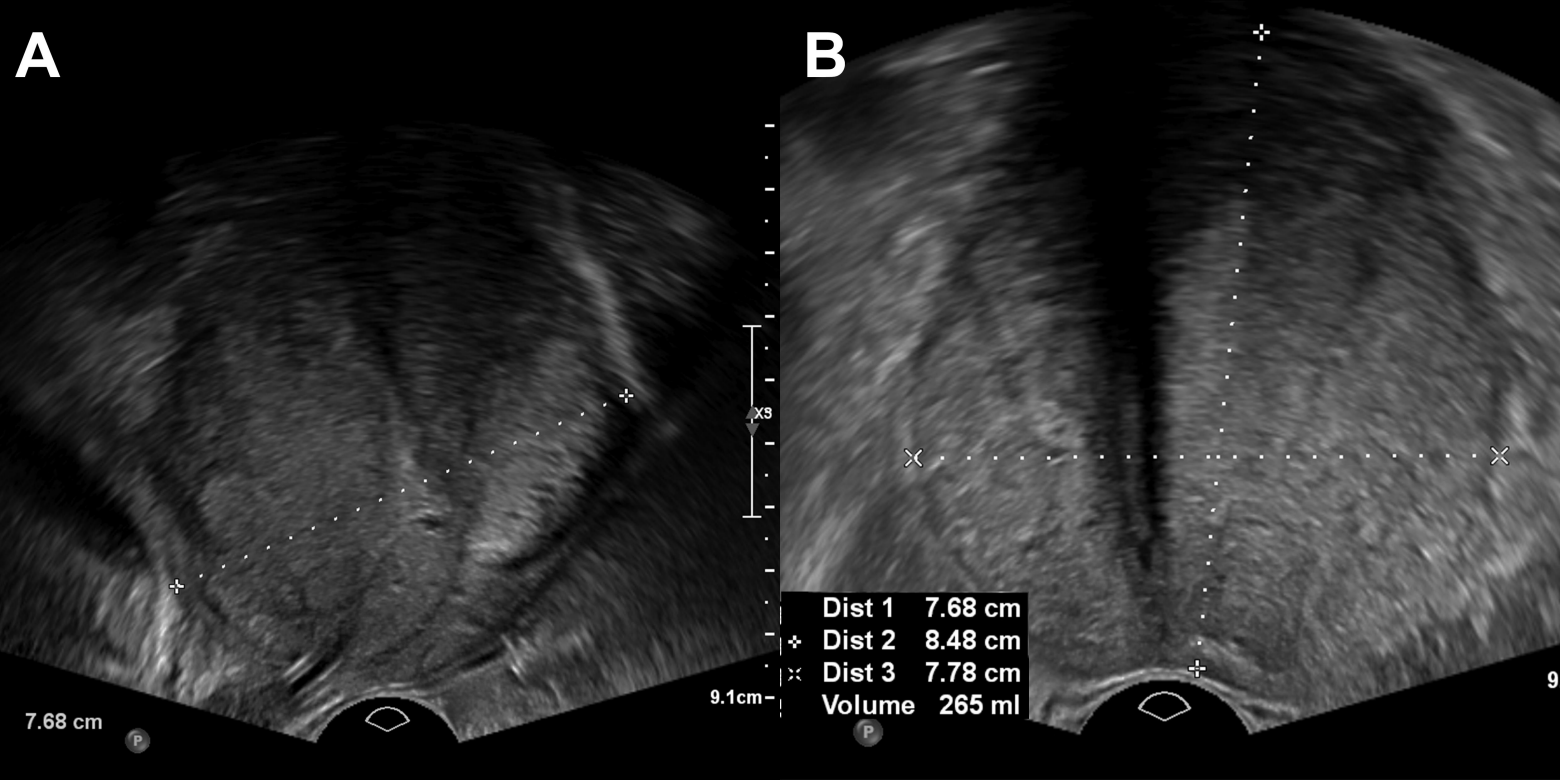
Transrectal ultrasound with sagittal (A) and axial (B) planes showing a volume of 265 mL.
